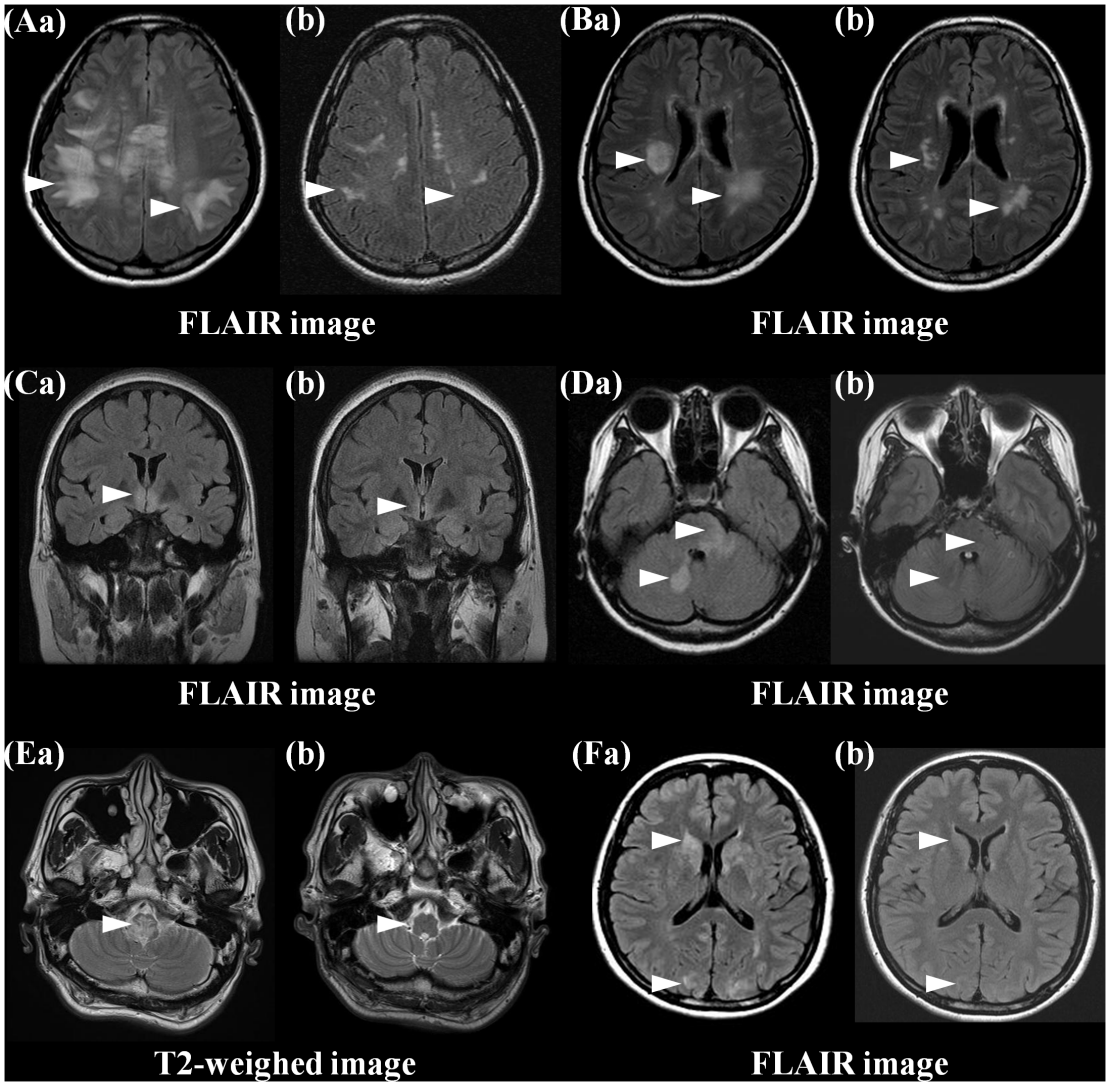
Most acute brain lesions in the white matter (Aa, Ba) showed a decrease in size and/or faded. Most remnant hyperintense lesions in hemispheric white matter on T2/FLAIR images were irregular in shape and located mainly in the subcortical/deep white matter (Ab, Bb). Acute hyperintense lesions in the bilateral hypothalamus (Ca) and around the fourth ventricle and cerebellum (Da, Ea), basal ganglia, and cortical/subcortical (Fa) on T2/FLAIR images resolved completely on follow-up brain MRI (Cb, Db, Eb, Fb).
